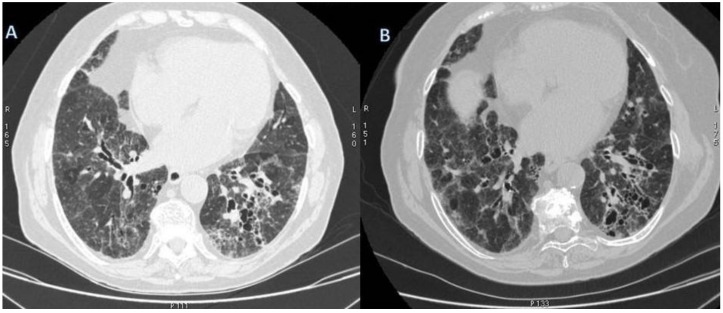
A 76-year-old female patient with GPA. Axial high-resolution CT shows irregular bronchial dilatation and interstitial lung alterations with interlobular and intralobular septal thickening and reticulations, predominantly located in the lower lobe (A). After six-months follow-up interstitial and bronchial are still recognizable (B).
